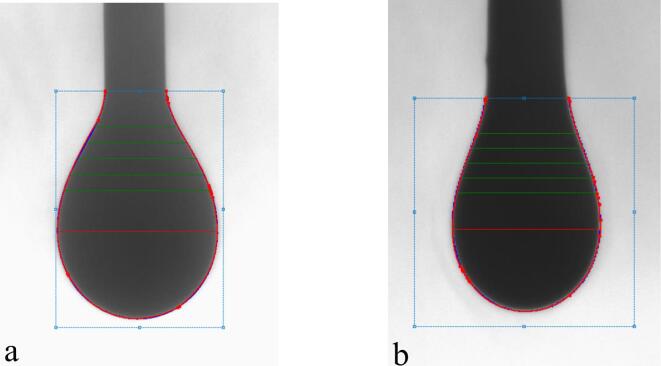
Results of Interfacial tension. (a) Image of the oil that has not been treated by ultrasound, σ_go_ = 11.02 mN/m; (b) Image of oil treated by ultrasound for 20 min, σ_go_ = 8.56 mN/m.
